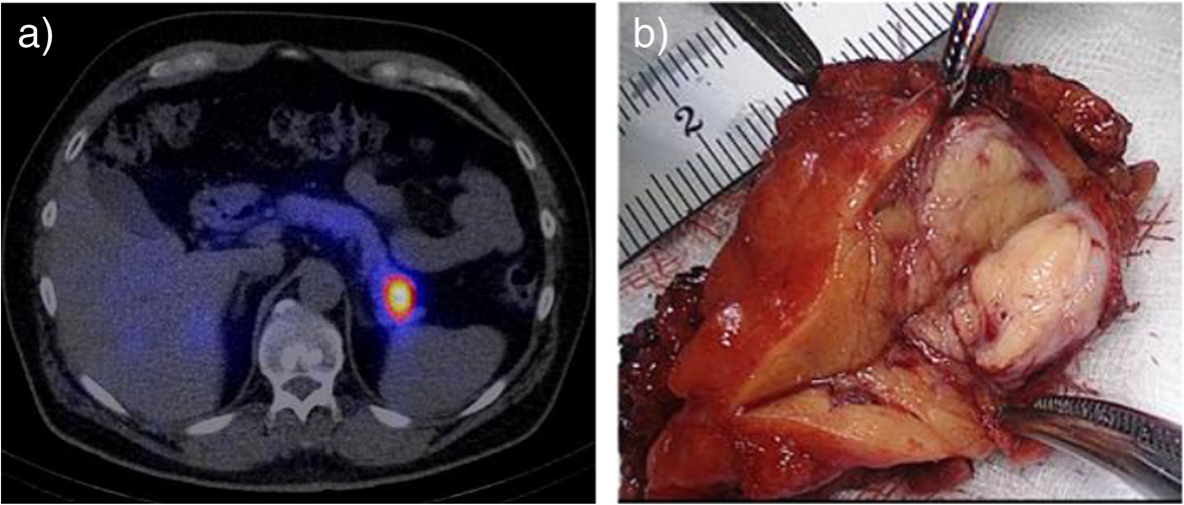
a) A large insulinoma of the pancreatic tail was detected with ^111^In-DOTA-exendin-4 SPECT/CT and b) resected laparoscopically (pancreatic tail resection).
